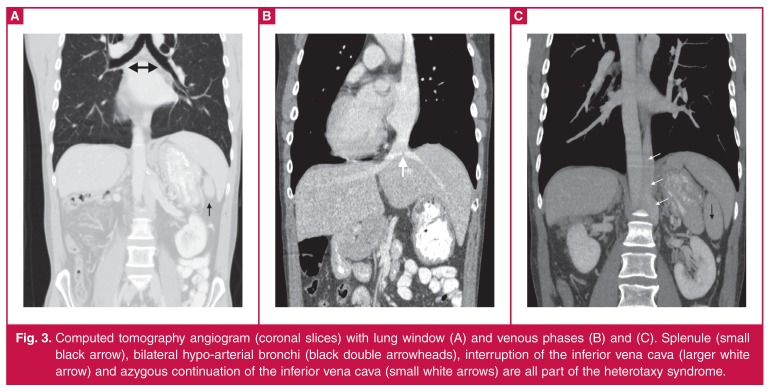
Computed tomography angiogram (coronal slices) with lung window (A) and venous phases (B) and (C). Splenule (small black arrow), bilateral hypo-arterial bronchi (black double arrowheads), interruption of the inferior vena cava (larger white arrow) and azygous continuation of the inferior vena cava (small white arrows) are all part of the heterotaxy syndrome.
